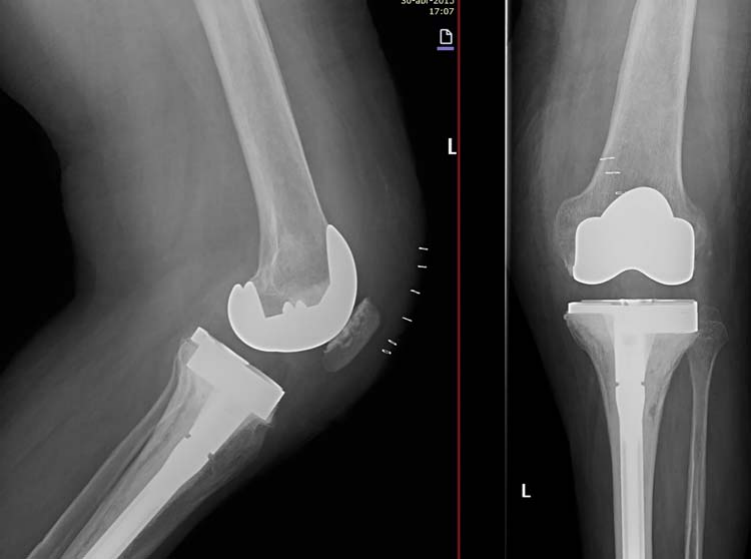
X-ray of the left knee showing reconstruction with 10-mm tibial lateral block and a tibial diaphyseal stem.
